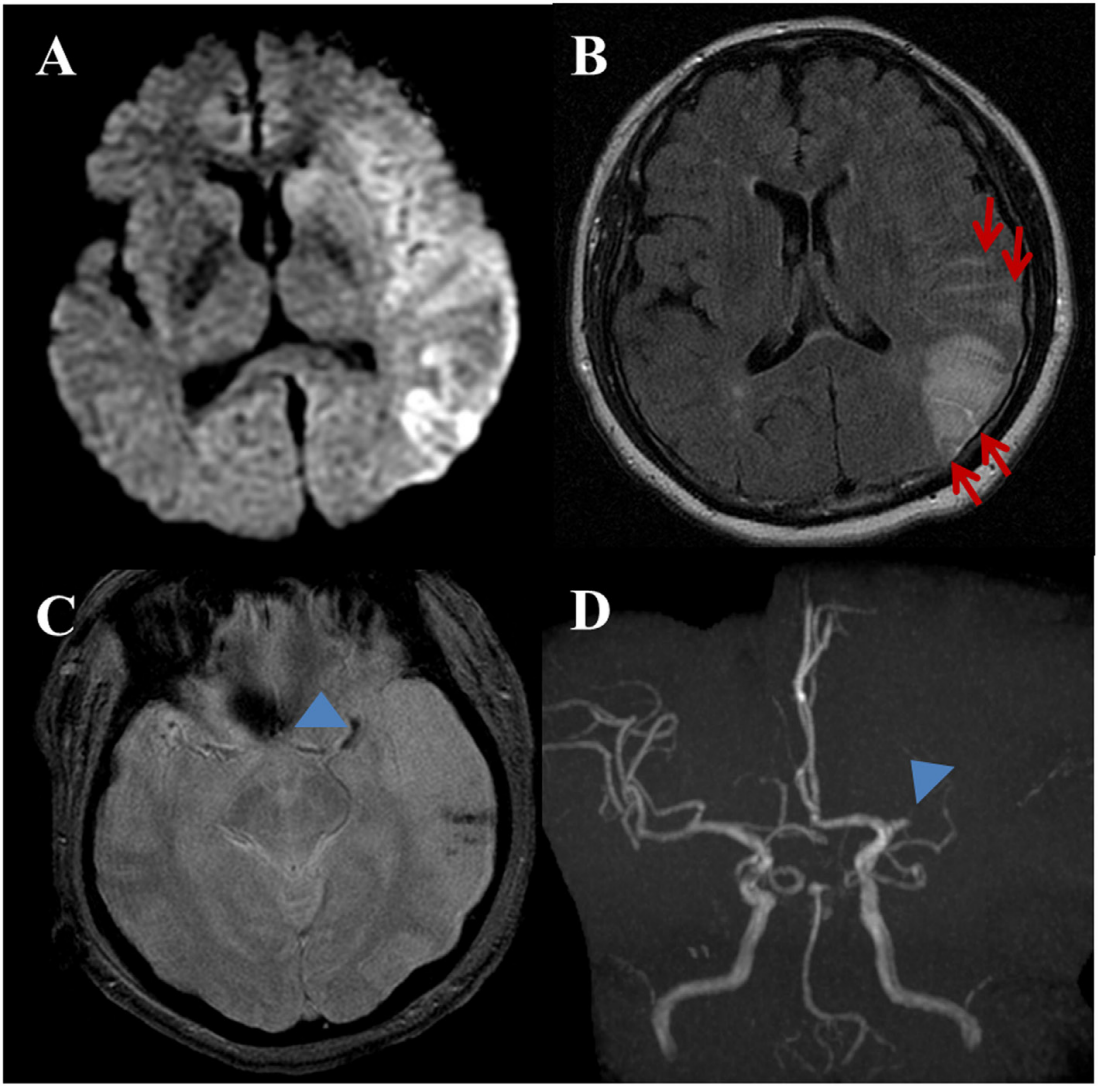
A 53-year-old female with a complaint of acute onset aphasia. Stroke due to cardioembolic. (A) DWI shows large diffusion restricted lesion on the left frontoparietotemporal lobes. (B) Post-contrast fluid-attenuated inversion recovery image shows sulcal enhancement along the left frontoparietal sulci (arrows) compatible with hyperintense acute reperfusion marker. (C) Gradient-echo imaging image shows blooming artifact at the left proximal MCA (arrowhead) indicating intravascular thrombus. Note, hemorrhagic foci of stroke area at the left temporal lobe. (D) Three-dimensional time-of-flight MRA shows occlusion of proximal M1 segment of left MCA (arrowhead). There is no stenoocclusive lesion in the cervical arteries (not shown).
